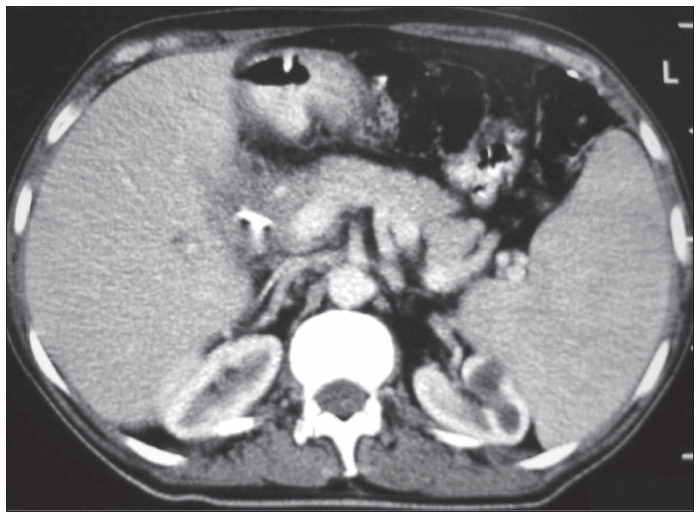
Computed tomography scan showing dilated intrahepatic ducts and splenomegaly, in a man with Hodgkin’s disease, four weeks after the placement of a plastic stent in a stricture of the bile duct.
